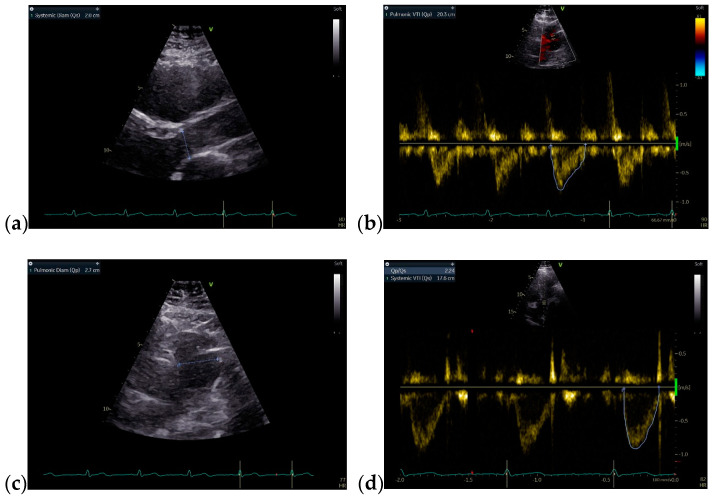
TTE on admission: (a) Parasternal long axis view measuring LVOT diameter = 2 cm; (b) Parasternal short axis view: Pulmonic VTI = 20.3 cm; (c) Parasternal short axis view: pulmonary artery trunk = 2.2 cm; (d) Apical 5 chamber view: Systemic VTI = 17.6 cm, Qp/Qs = 2.24; Abbreviations: TTE—transthoracic echocardiography; LVOT—left ventricle outflow tract; VTI—velocity-time integral.
